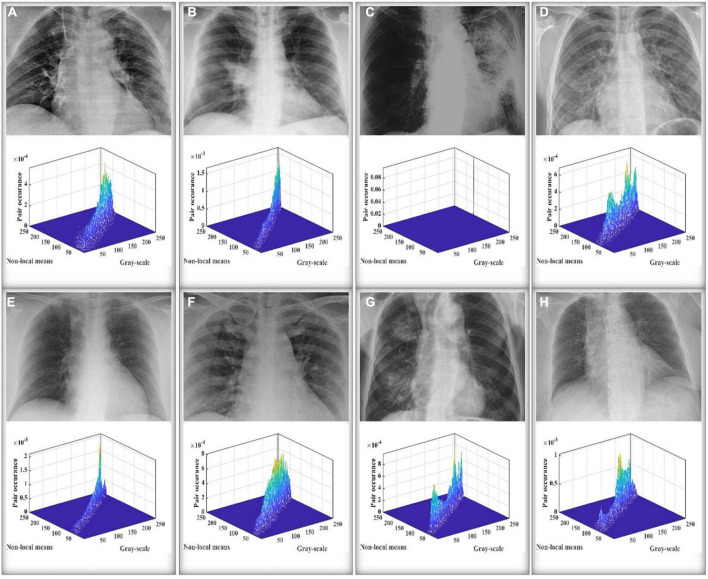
The COVID-19 X-ray images used in the segmentation experiment.
